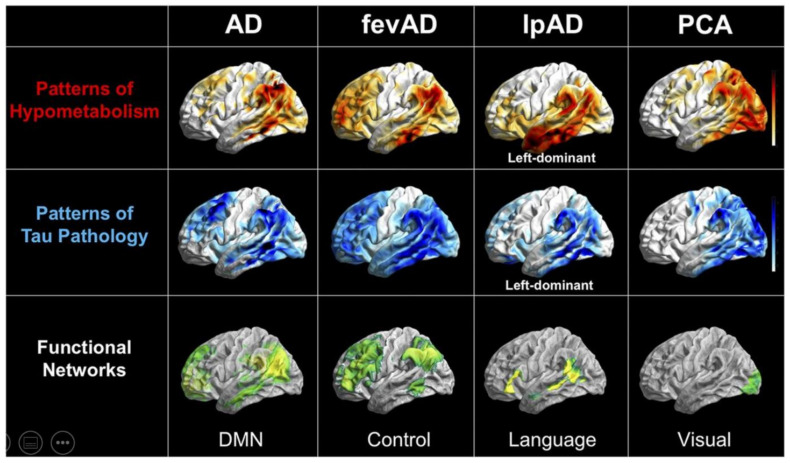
Conceptual depiction of a partial correspondence between hypometabolism (FDG PET), tau PET and functional networks in typical AD (left) and three subtypes, frontal-executive variant AD (fevAD), logopenic progressive aphasia (lpAD) and PCA. This figure was originally published in JNM [153]. Reproduced with permission and courtesy of Merle Hoenig, University of Cologne, Germany.
